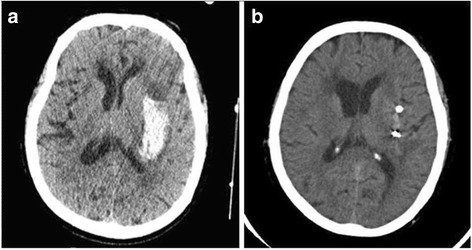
Hematoma changes after minimally invasive surgery. a The hyper-density on brain CT indicates pre-operative ICH in the left basal ganglion. b The ICH was removed by the minimally invasive surgery
